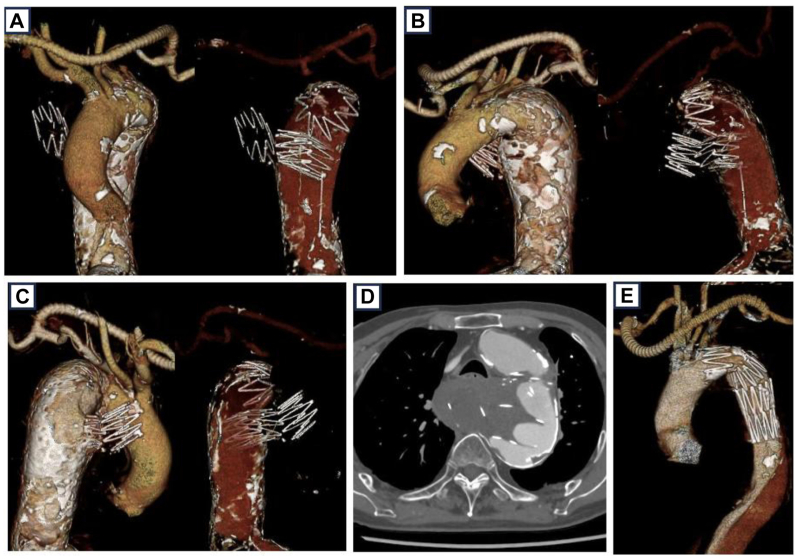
Computed tomography (CT) findings before and after stent graft migration. (A-C) Three-dimensional reconstructed CT images showing the thoracic aorta from different angles. The *right panels* in each pair depict the same views with the stent graft struts rendered visible to demonstrate the configuration and marked distal migration of the fractured components protruding into the posterior mediastinum. (D) Axial contrast-enhanced CT image demonstrating the migrated stent graft within the posterior mediastinum, compressing the esophagus. (E) Three-dimensional CT image obtained immediately after the initial debranching thoracic endovascular aortic repair (TEVAR) performed 16 years earlier, showing the intact custom-made stent graft in the distal aortic arch.
